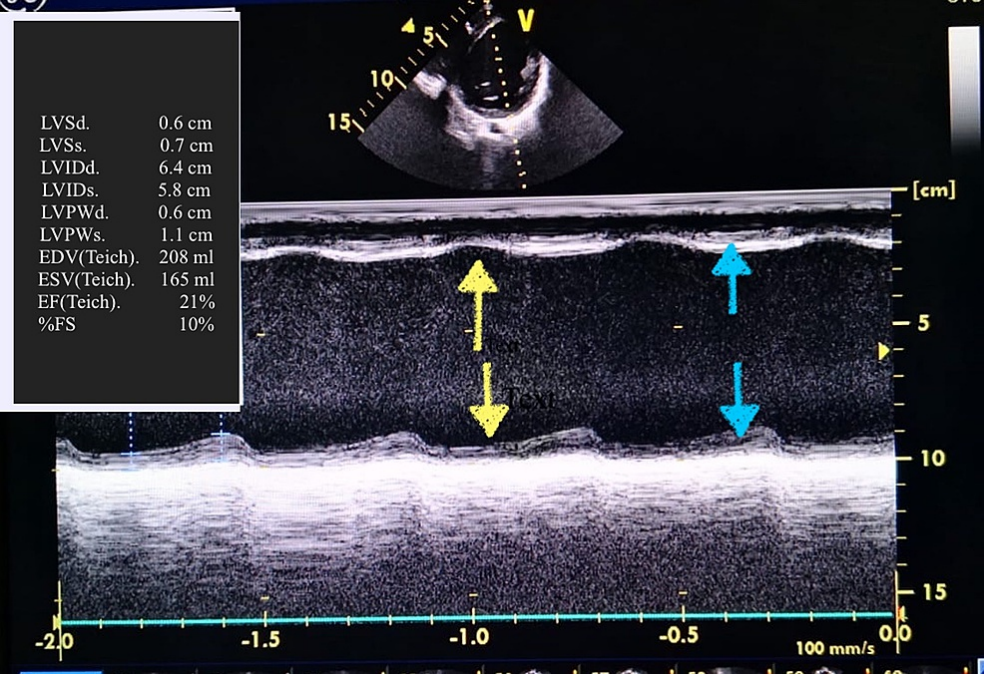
Echocardiogram M mode of left ventricle showing severe depression of myocardial contractility (blue arrows showing weak contractility) with wall asynchrony (yellow arrows showing incoordination between the wall muscles) and LV EDV 208 mL and LV ESV 165 mL with estimated ejection fraction of 21% and fraction of shorting of 10% LV EDV: left ventricular end-diastolic volume; LV ESV: left ventricular end-systolic volume; M mode: motion mode
